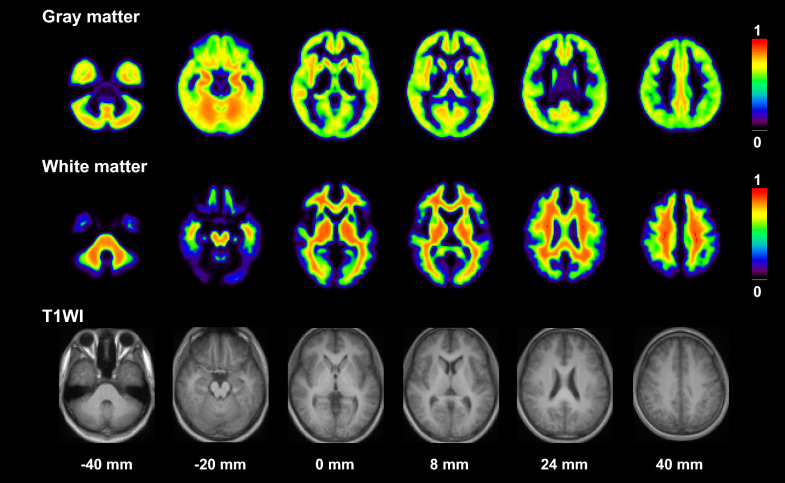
Averaged gray and white matter images extracted from anatomically standardized MR images. All images are transaxial sections parallel to the anterior–posterior commissure (AC–PC) line. The slice positions are − 40, − 20, 0, 8, 24 and 40 mm from the AC–PC line
